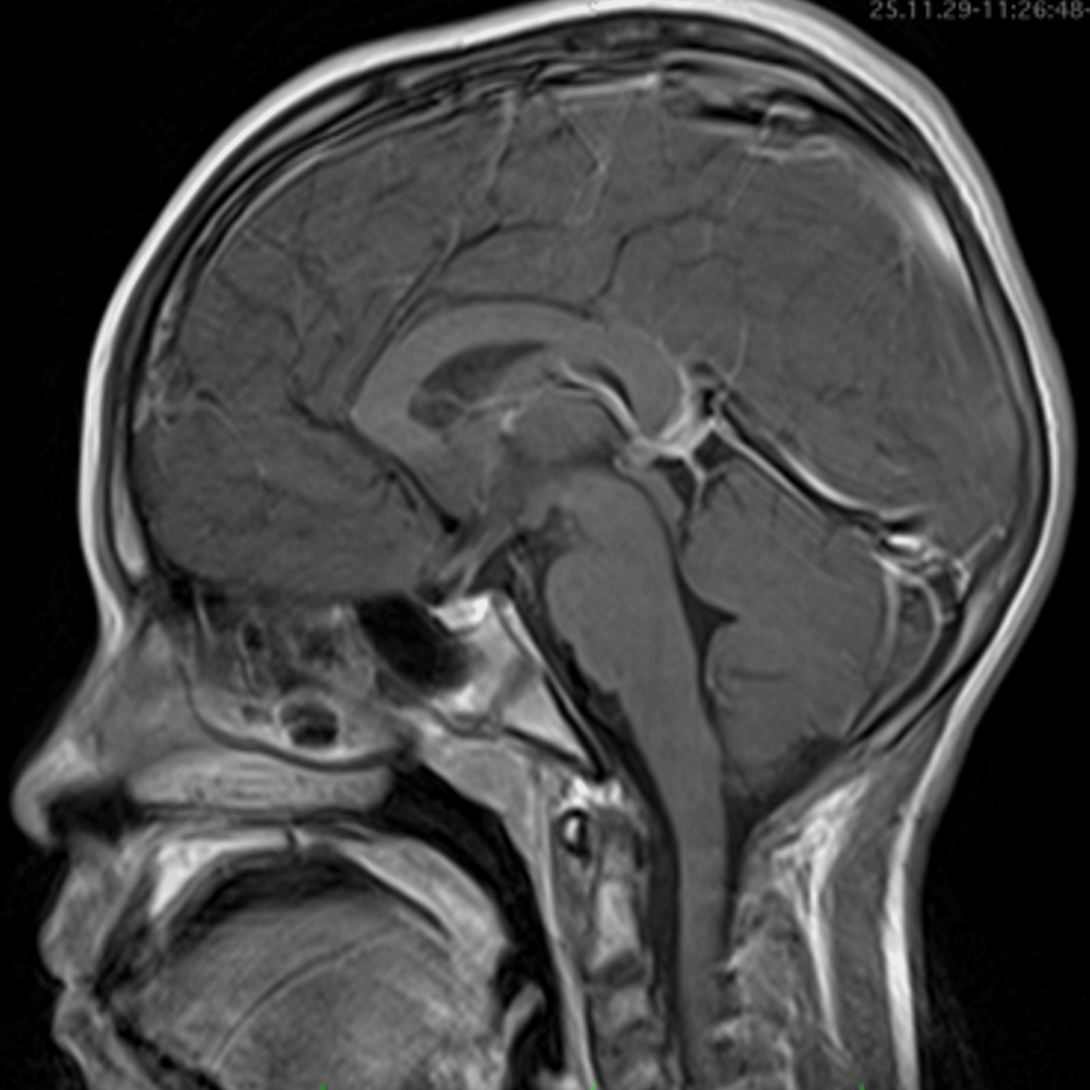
Postoperative follow-up. Postoperative magnetic resonance imaging showing complete surgical resection.
